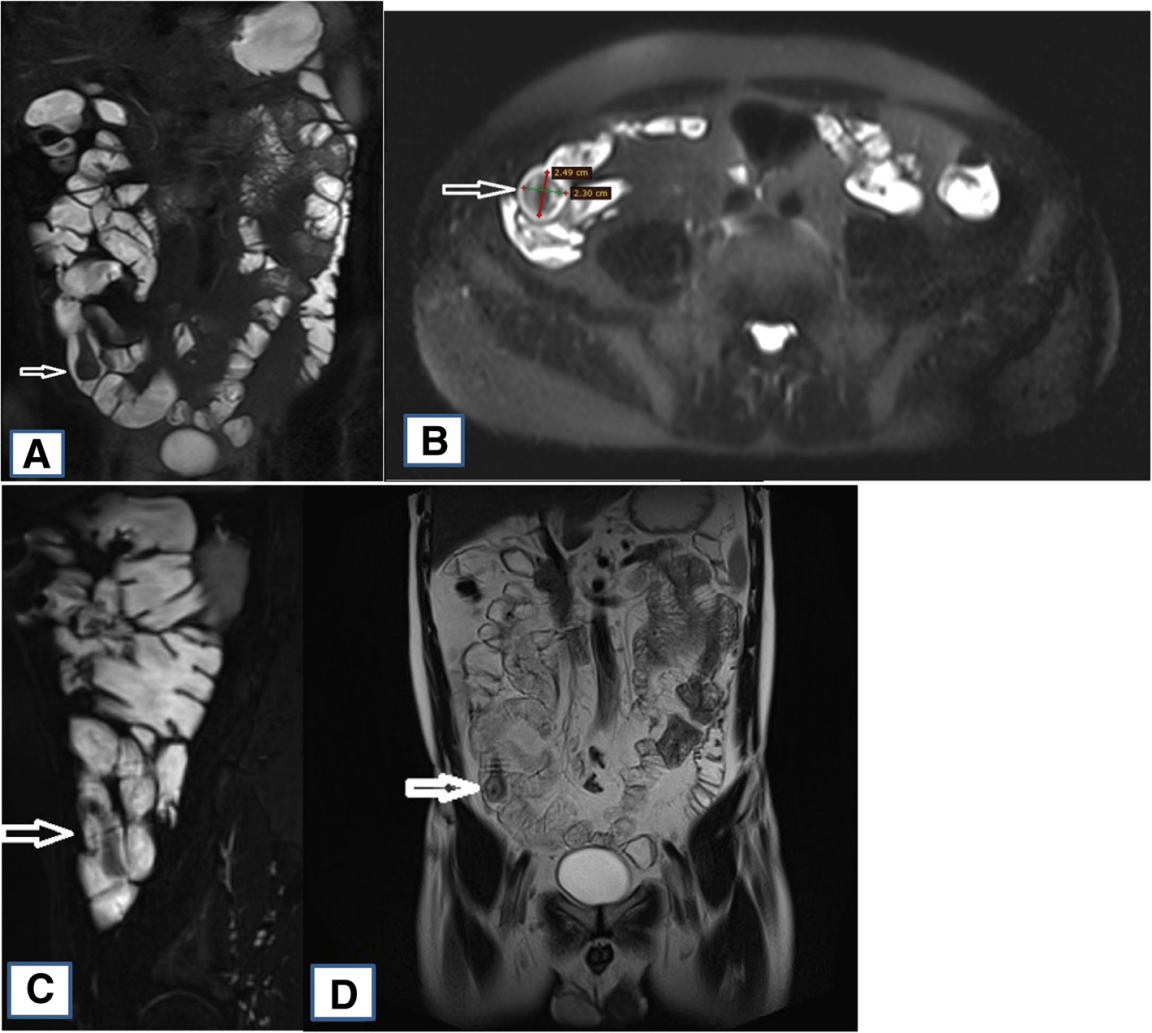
MR enterography: large pedunculated polyp. a Coronal T2-weighted image, mannitol as a luminal contrast, no intravenous contrast. b Axial T2-weighted image, mannitol as a luminal contrast, no intravenous contrast (polyp size 2.5 × 2.3 cm). c Sagittal T2-weighted blade fat saturation (fs) image, mannitol as a luminal contrast, no intravenous contrast (good view of long pedunculus). d Coronal T1-weighted blade image, mannitol as a luminal contrast, no intravenous contrast. e Coronal T1-weighted vibe fs image, intravenous contrast
